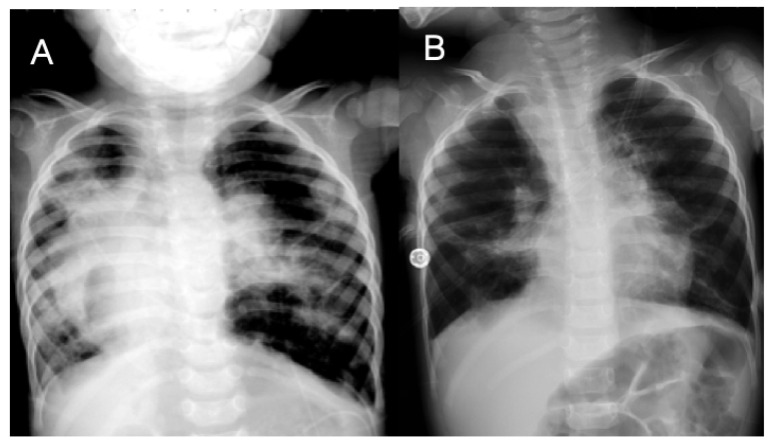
An extremely preterm male infant was diagnosed with bronchopulmonary dysplasia (BPD) and pulmonary hypertension. Chest radiography at the age of 3 years demonstrated dextrocardia, widespread coarse interstitial markings, atelectasis, and regions of hyperexpansion (A). A preterm female infant was diagnosed with BPD and pulmonary hypertension. Her chest radiography at the age of 2 years also showed the coarse interstitial markings, atelectasis, and regions of hyperexpansion (B) typical of BPD.
